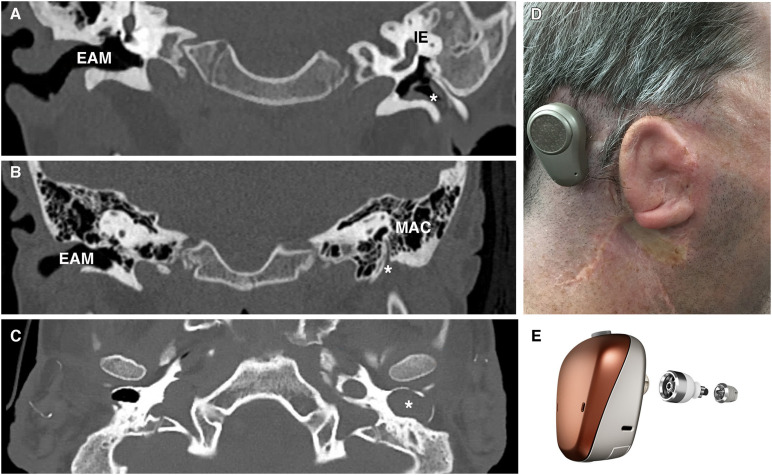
Patient photograph and scans of congenital aural atresia. (A) Congenital aural stenosis. Coronal CT scan shows a normal, patent right ear canal and a stenotic left ear canal marked by *. (B) Complete congenital aural atresia. Coronal CT scan shows a normal ear canal on the right and an atretic plate on the left with no ear canal. (C) Congenital aural stenosis and cholesteatoma. Axial CT scan of a patient with congenital aural stenosis and left-sided cholesteatoma. The bony ear canal has been widened and remodeled by the collecting keratin (marked by *). (D) Photograph of a patient with Treacher-Collins syndrome and complete aural atresia of the left ear canal. The patient has been fitted with an osseointegrated hearing device visible behind the pinna. (E) Example of an osseointegrated device. Computer-generated image of a BAHA Connect (Image courtesy of Cochlear Bone Anchored Solutions AB, ©2020). EAM, external auditory meatus; IE, inner ear; MAC, mastoid air cells.
